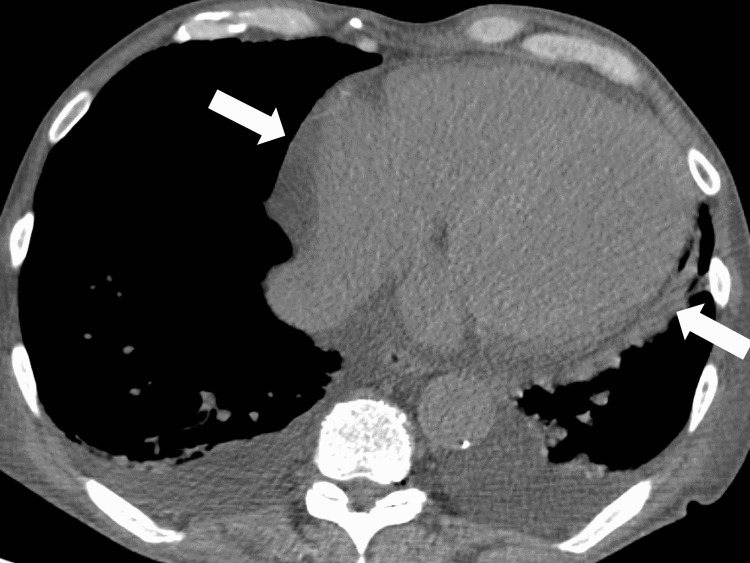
Pericardial effusion on the initial computed tomography
